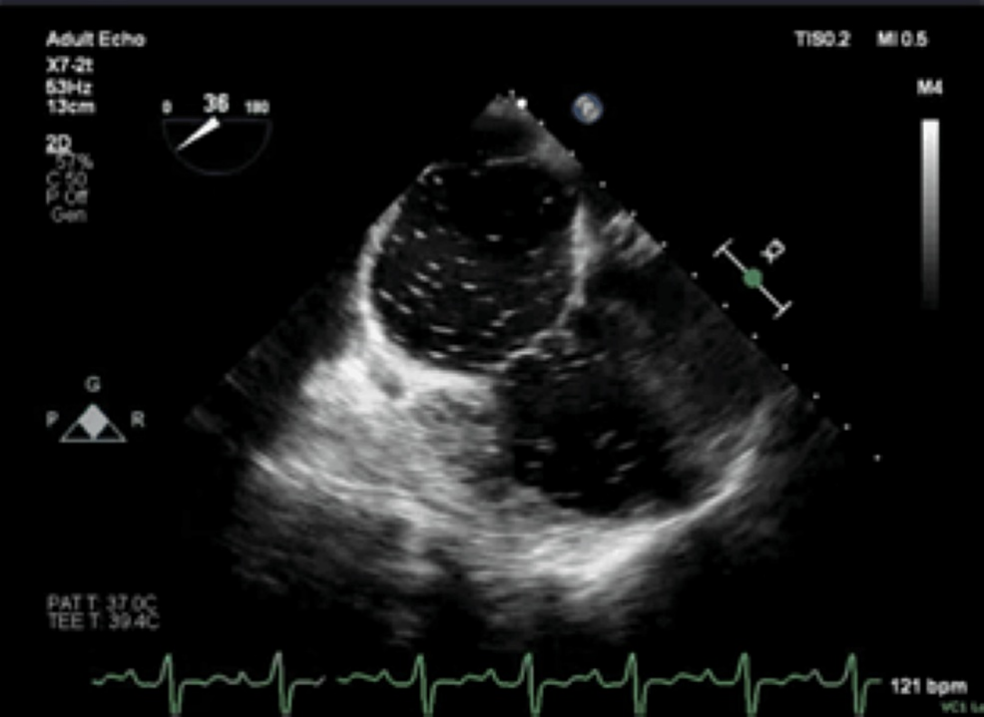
Second TTE with a successful agitated saline study revealing an interatrial septal defect with biatrial shunting TTE: Transthoracic echocardiogram
